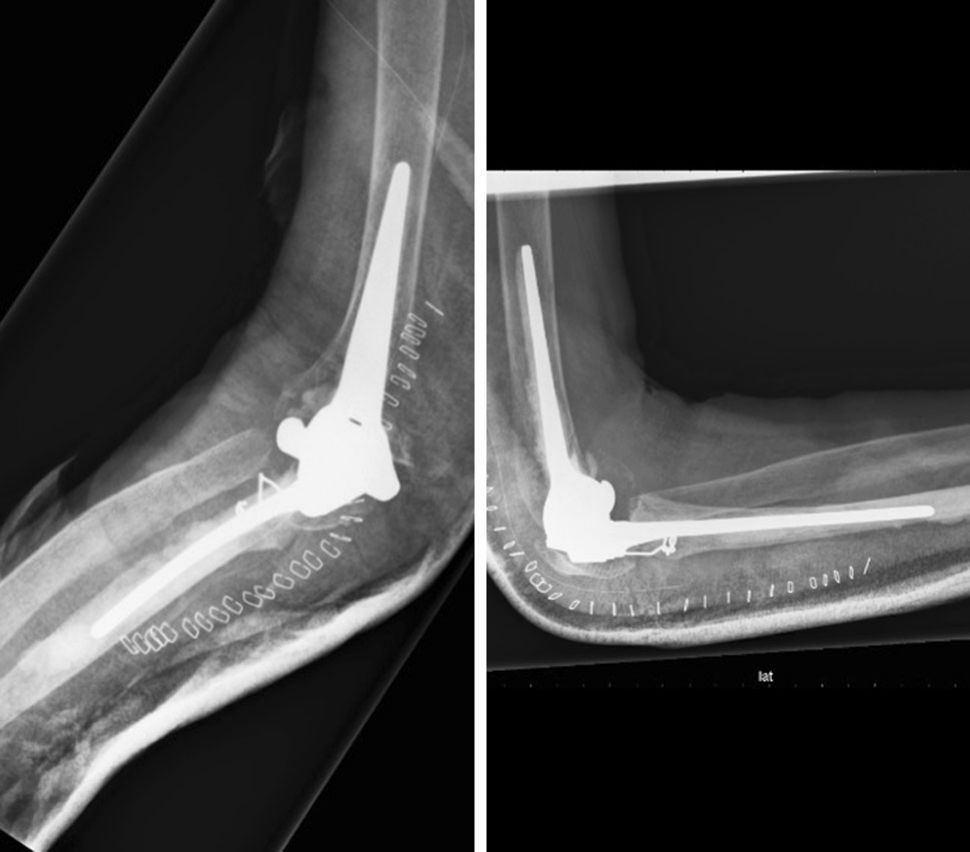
GSB III Elbow Prosthesis; this prosthesis does not have a humeral anterior flange. On an anterior–posterior X-ray, the GSB III Elbow Prosthesis is recognizable because of the large joint surface of the humeral component
